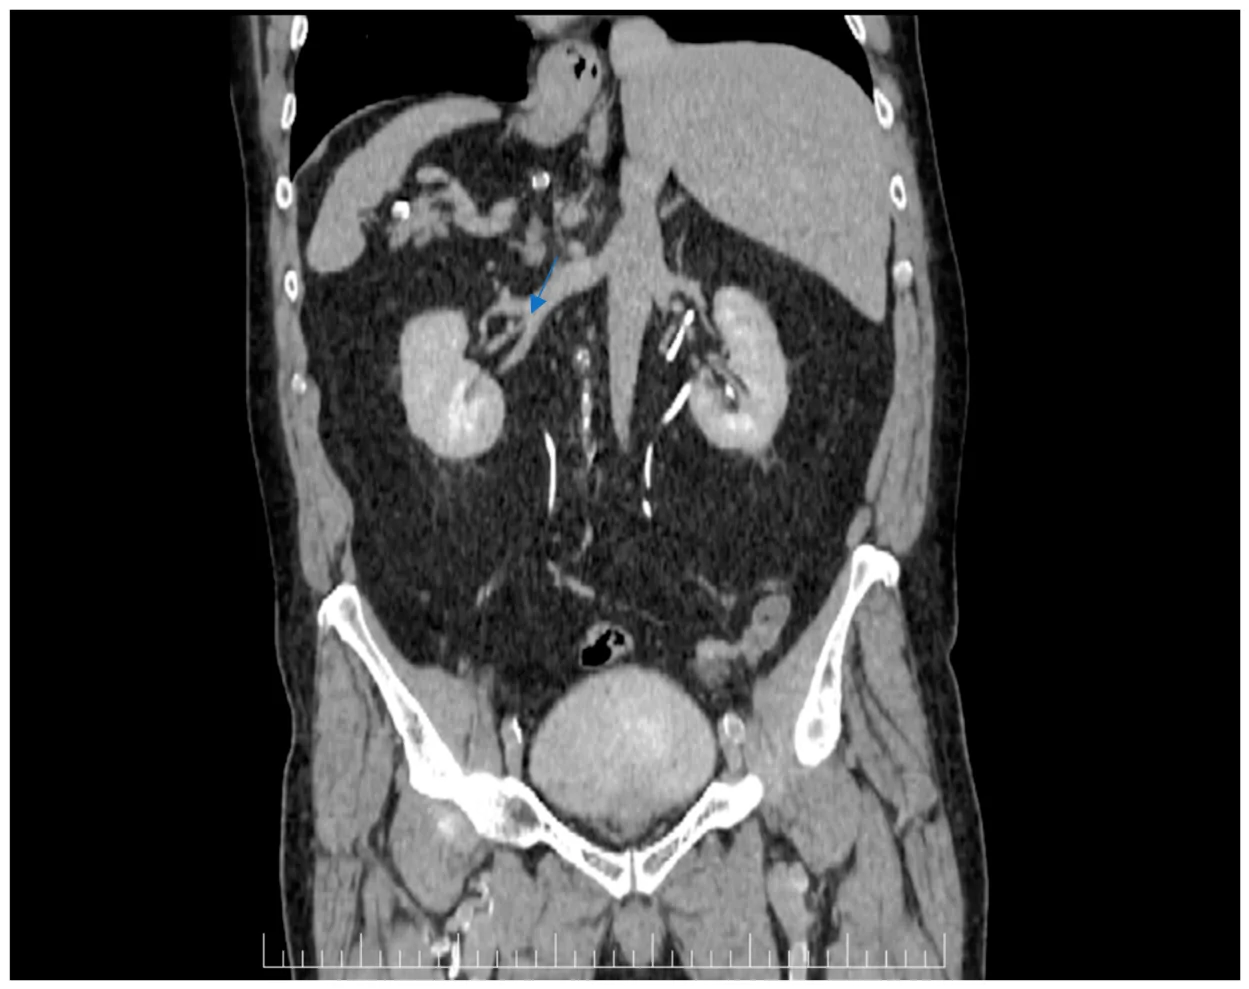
Contrast-enhanced computed tomography (CT) image of the abdomen and pelvis in the coronal plane, showing a right-sided duplicated pyeloureteral system with distal fusion near the vesicoureteral junction resulting in a single ureteral insertion into the bladder (arrows). No hydronephrosis, ureteral dilatation, ectopic insertion, or obstructive uropathy was observed.
